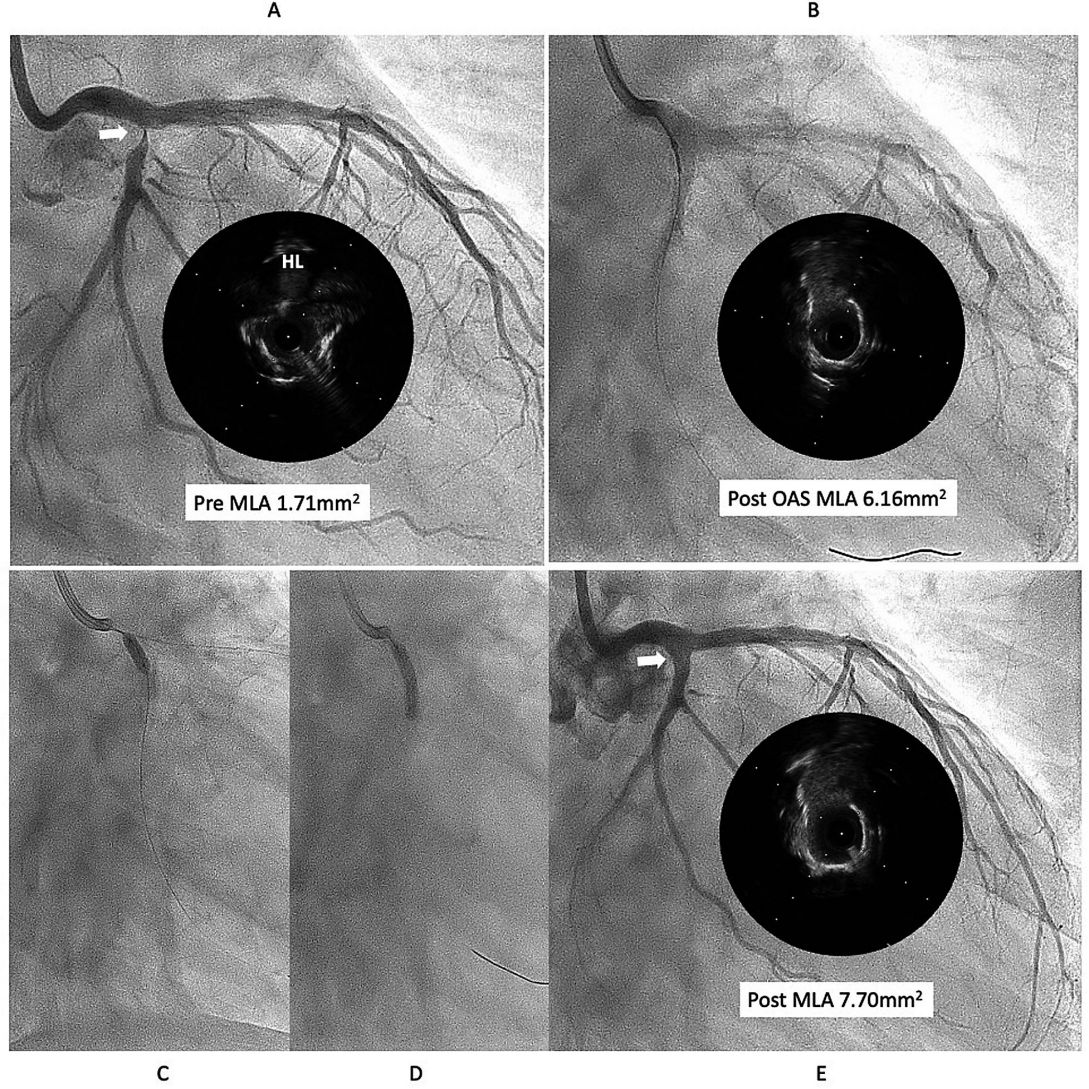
First intervention for left circumflex (LCX) ostial lesion (white arrow). (A) The intravascular ultrasound (IVUS) showed that calcified plaque is protruding into the lumen and surface is relatively smooth, that indicates the lesion is a non-eruptive calcified nodule (CN). (B) Diamondback micro crown was used to reduce the calcified tissue, with 7 runs at low speed and 6 runs at high speed. IVUS after ablation demonstrated a significant cutting effect, and lumen was well enlarged. (C,D) lesion was dilated using Wolverine cutting balloon 3.0 × 10 mm, followed by SeQuent Please 3.5 × 20 mm. (E) Final angiogram and IVUS showed good lesion dilation (white arrow). HL indicates high-lateral branch. MLA indicates minimum lumen diameter. OAS indicates orbital atherectomy system.
